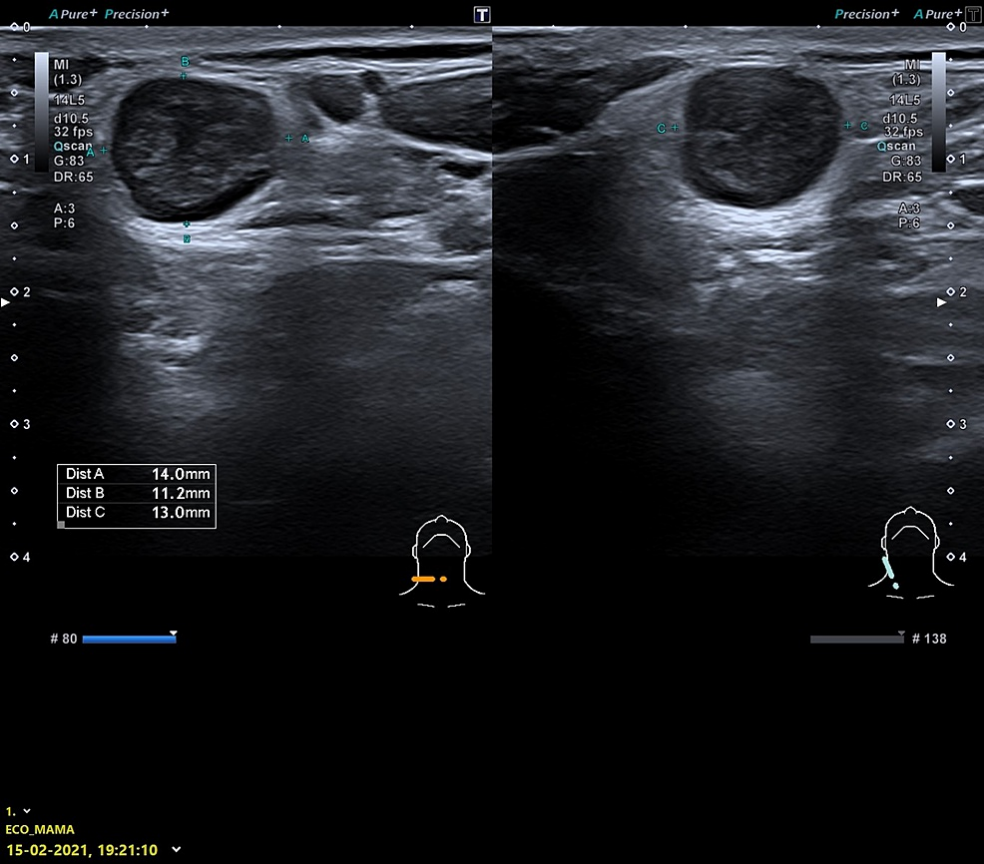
Cervical ultrasonography - February 15, 2021 The image shows one of the adenopathies with increased sphericity index, hypoechogenic, without defined hilum, and 14 x 11 mm in size
